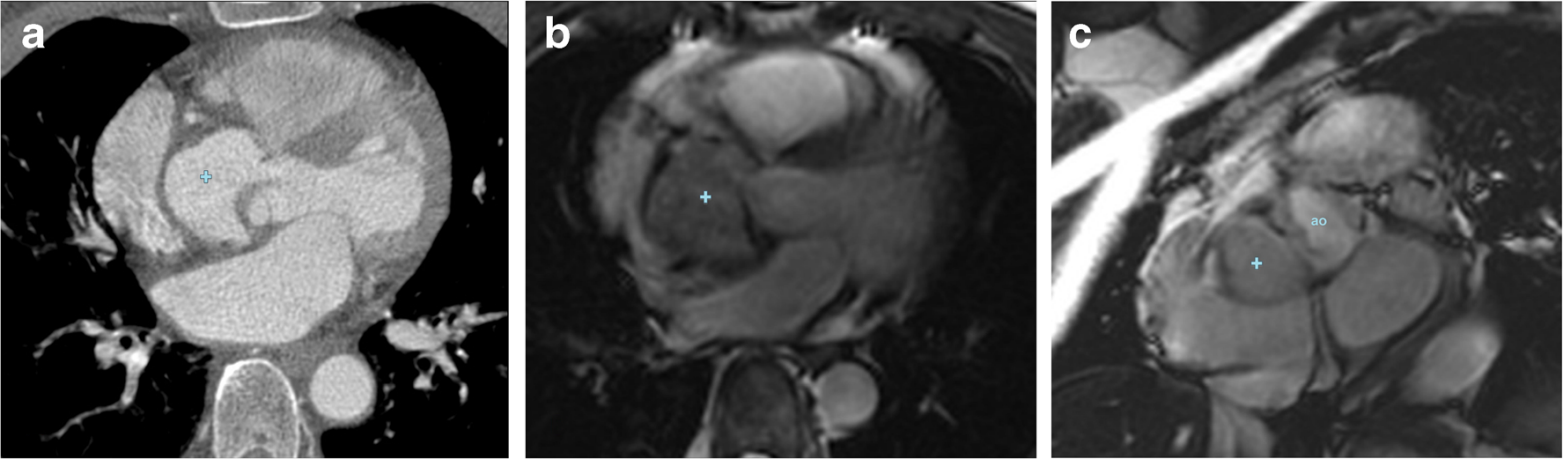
54-year-old man who presented with intermittent syncope. CT axial image MPR (a) shows a large IVMS aneurysm (+) bulging and displacing the right ventricle and atrium. MRI (bSSFP sequences) in the axial (b) and short axis (c) planes reveal a small necked large aneurysm (+) outpouching below the LVOT (ao)
